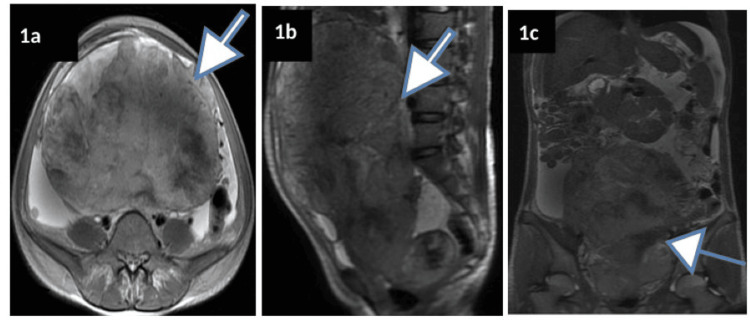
Preoperative MRI revealing a large, relatively well-circumscribed soft tissue mass (arrows) measuring 23 × 18.5 × 12 cm centered in the uterine corpus The mass is well visualised on the axial (a), sagittal (b), and coronal (c) views, highlighting its extent and invasive nature. It exhibits irregular margins and demonstrates infiltration into the myometrium and parametrium while displacing and compressing the adjacent pelvic structures. However, there was no apparent extension to the anterior rectal wall and posterior bladder wall.
